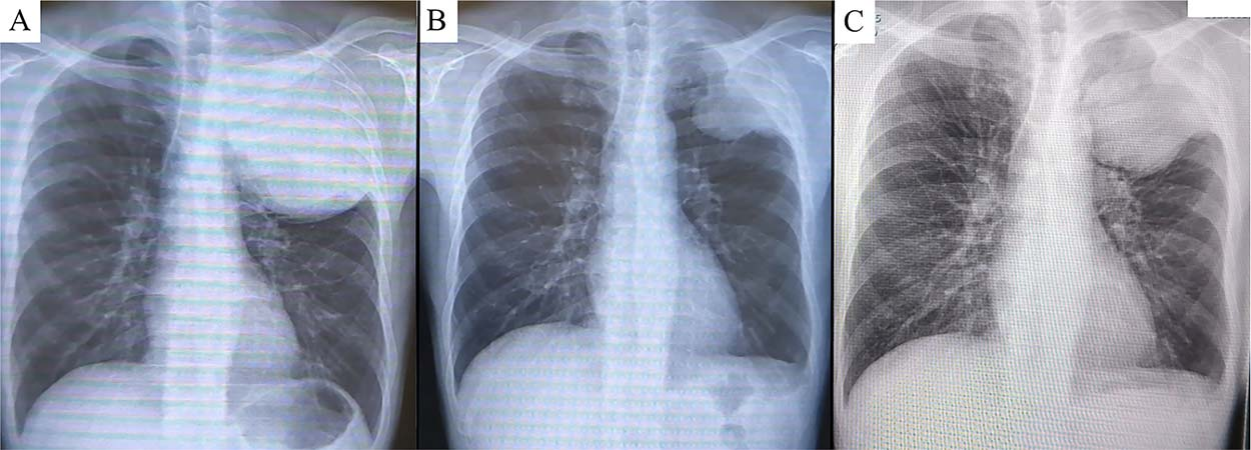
Chest X-ray prior to treatment revealed a large mass extending from the left lung apex to the upper lung field (A), which markedly decreased in size following immunotherapy (B). However, during the 4-week interval between the end of immunotherapy and surgery, the tumor demonstrated regrowth (C).
